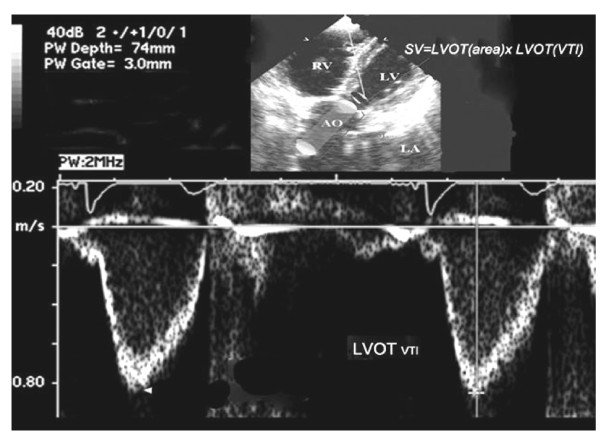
Blood leaves the left ventricular outflow tract (LVOT) as a cylinder (aorta shown in *red*). The volume of the cylinder is equal to the stroke volume (*SV*). In order to calculate SV, the echocardiographer must obtain the diameter of the LVOT and velocity time integral (VTI) of the blood measured at the same exact location. *Ao *= Aorta, *LV *= left ventricle, *RV *= right ventricle, *LA *= left atrium.
